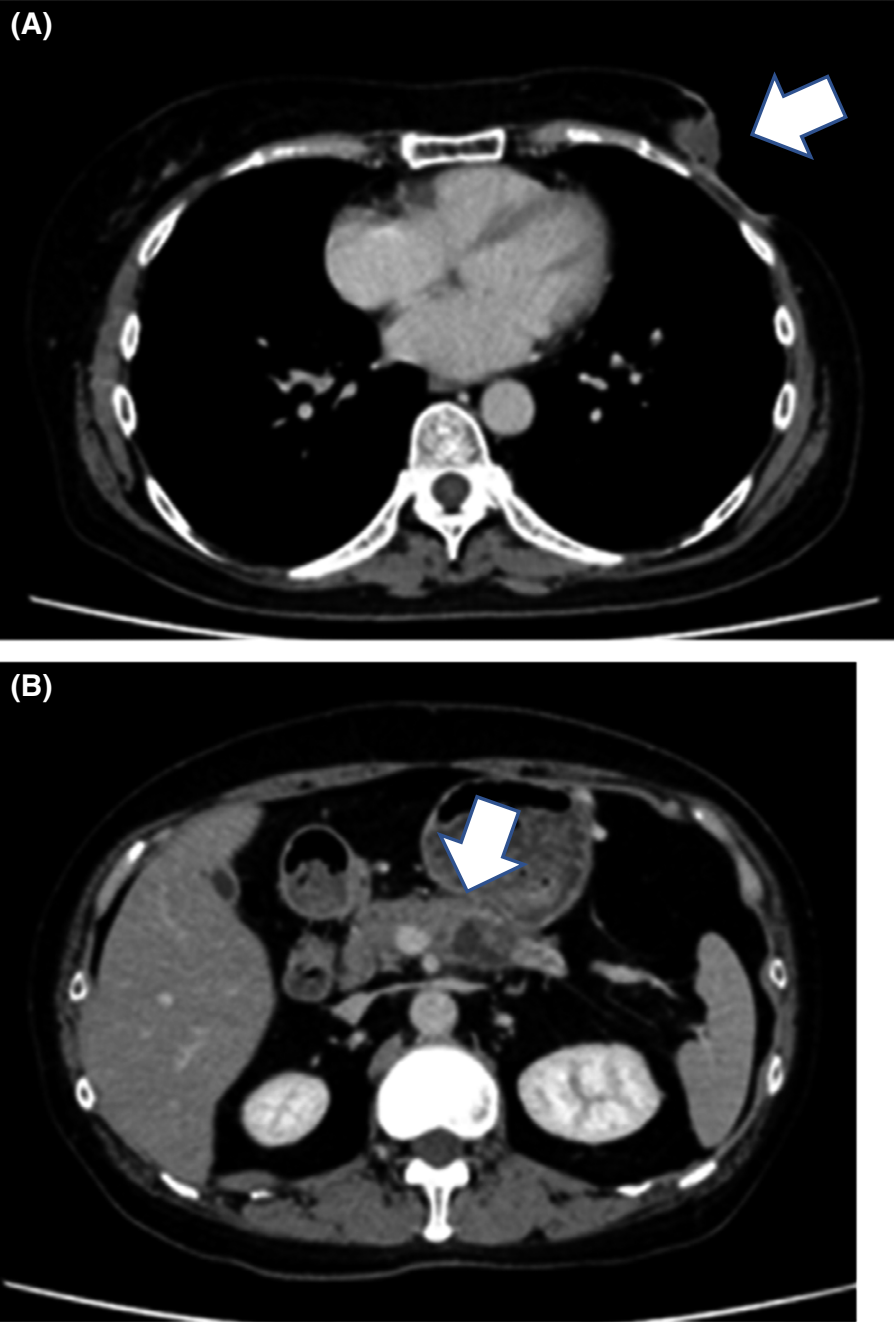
Computed tomography after 4 cycles of paclitaxel + bevacizumab. A, Shrinkage of the tumor in the left breast (Arrow). B, Increase in the size of pancreatic tumor, which is seen infiltrating the superior mesenteric artery (Arrow)
